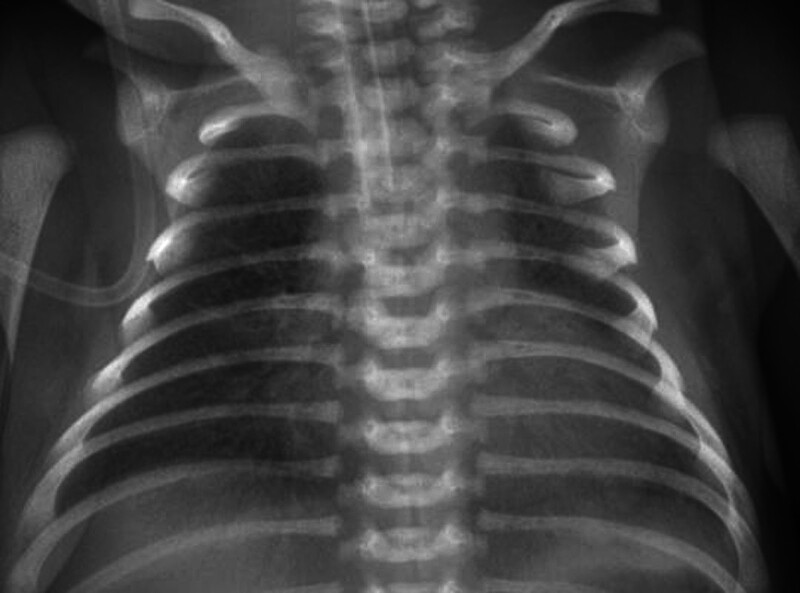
The admission chest X-ray examination shows a significant decrease in the translucency of both lungs, with “white lung”-like changes. The image shows the enhanced texture of both lungs, patches of faint shadows in both lungs, the whole heart shadow, and blurred diaphragmatic surfaces.
